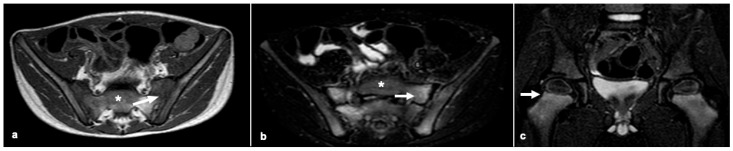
Magnetic resonance images in a four-year-old boy with scurvy. (a) An axial pcst-contrast T1 image shows bilateral, symmetric multifocal water-like signals in the ilium and sacrum (arrow); (b) An axial short tau inversion recovery (STIR) demonstrates sacroiliac contest-enhancement (arrow). In panels (a,b), (*) indicates bone marrow with normal appearance in the sacral region. (c) A coronal STIR image showing bilateral hyperintensities in the proximal femoral metaphysis (arrow).
